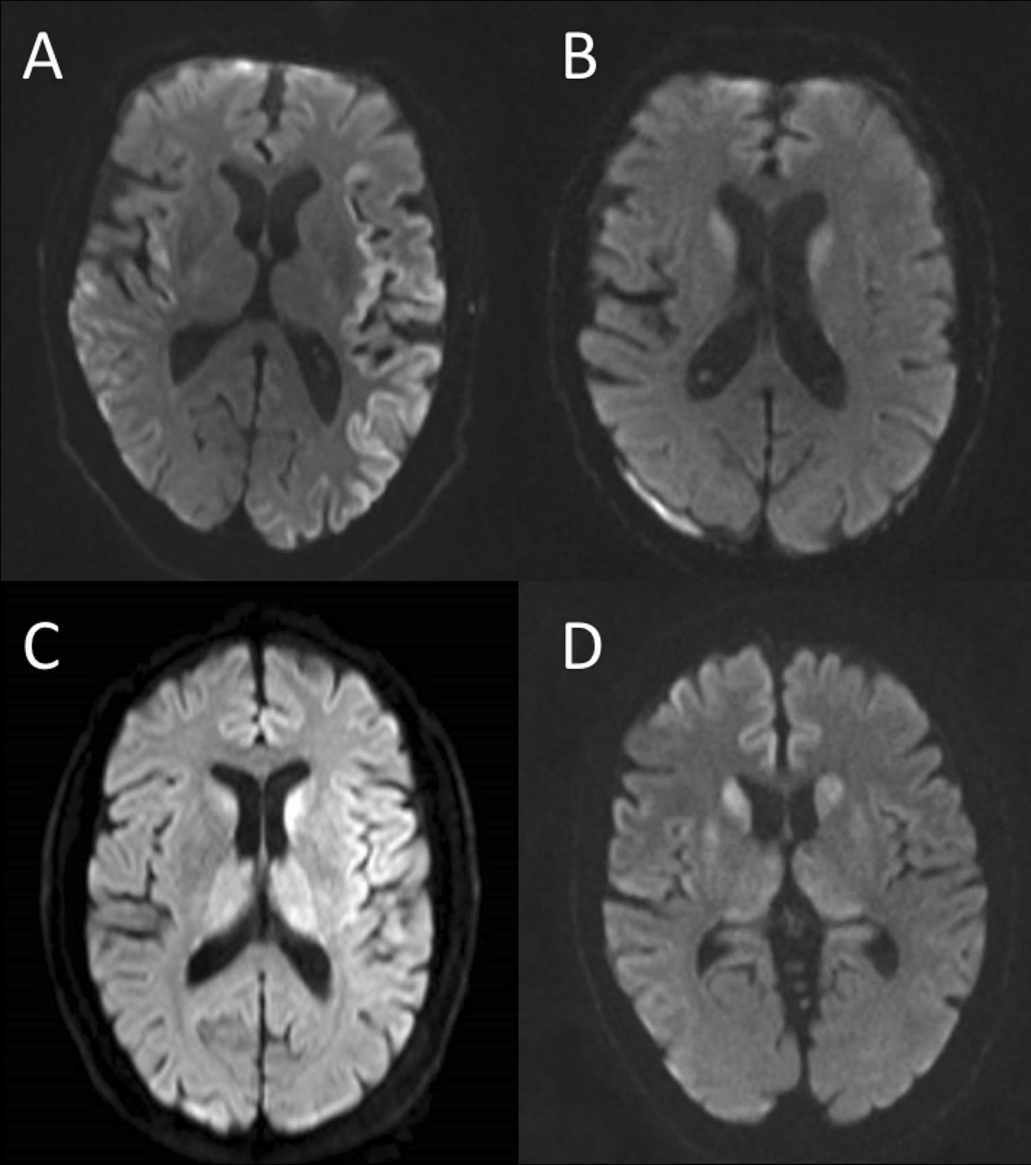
Initial MRI scans from four separate cases, not reported by referring centres as being suspicious for CJD. Image A displays significant cortical involvement which was noted by the radiologist, with differentials of seizure or hypoxia listed. Image B shows high intensity change in the striatum, which was not documented on the report. Images C and D demonstrate high intensity changes in all three regions that are characteristically affected in CJD (cortex, thalamus and striatum). These changes were missed in the report for Image C, whilst the report for image D mentioned abnormal signal in the basal ganglia only, and suggested differentials including hypoglycaemia, hypoxia, mitochondrial and metabolic causes
